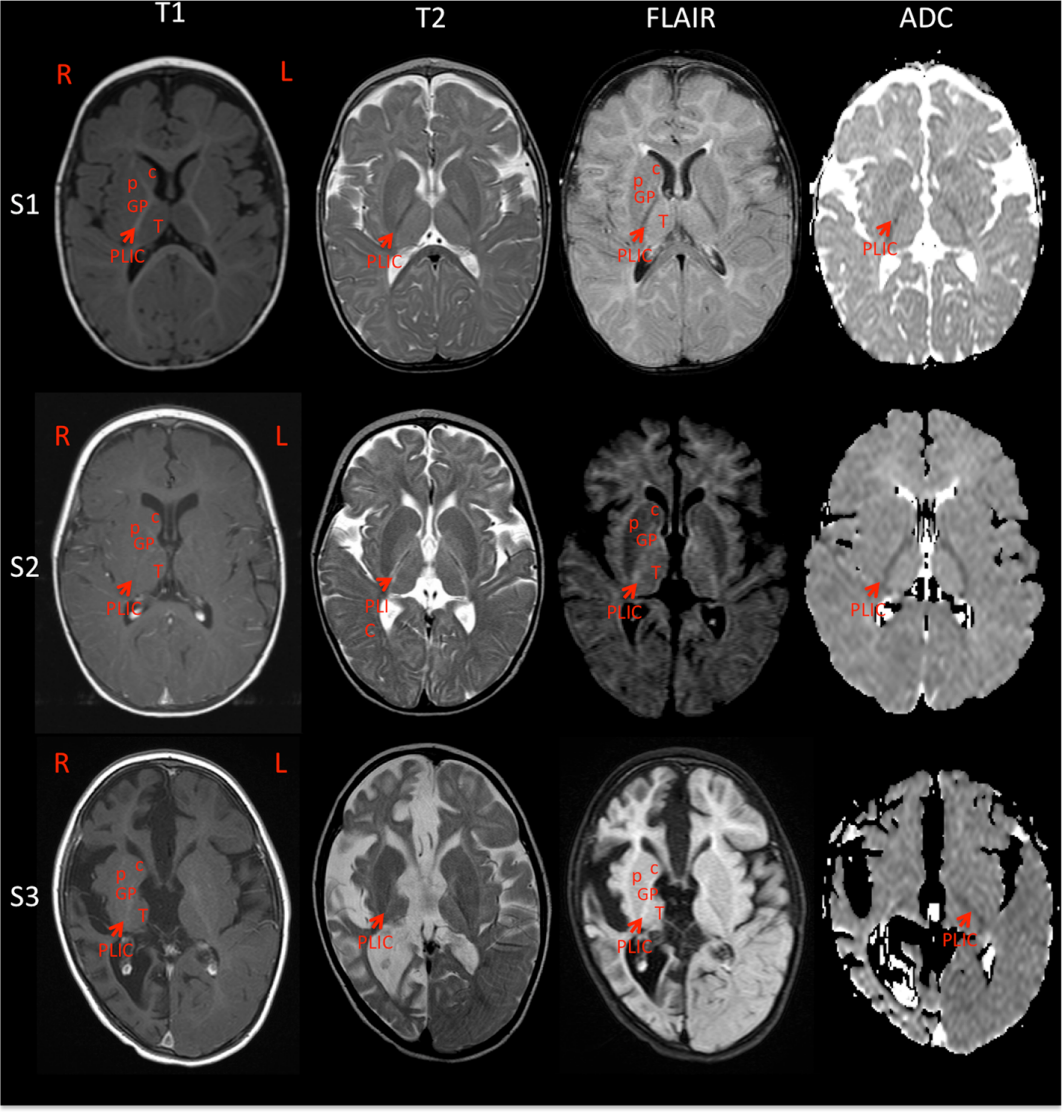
Cranial MRI shows a consistent pattern of hypomyelination across ABAT patients. MRI of Subject 1 (S1) at age 9 months shows extensive T2 hyperintensity in the deep white matter that lags ~5 months behind the patient’s chronological age, suggesting diffuse mild hypomyelination. However, the anterior limb of the internal capsule is hyperintense on T1, and partially hypointense on T2, with a non-restricted ADC map. In contrast, while Subjects 2 (S2) and 3 (S3) are older at 13 and 17 months, myelination in the posterior limbs of the internal capsules is less complete and associated with restricted diffusion on ADC, suggestive of more severe hypomyelination. Additionally, Subjects 2 and 3 exhibit diffuse yet mild cerebral atrophy. Subject 3 also exhibits asymmetry with disproportionate right sided atrophy. C: caudate, P: putamen, GP: globus palidus, T: thalamus, PLIC: posterior limb of the internal capsule
